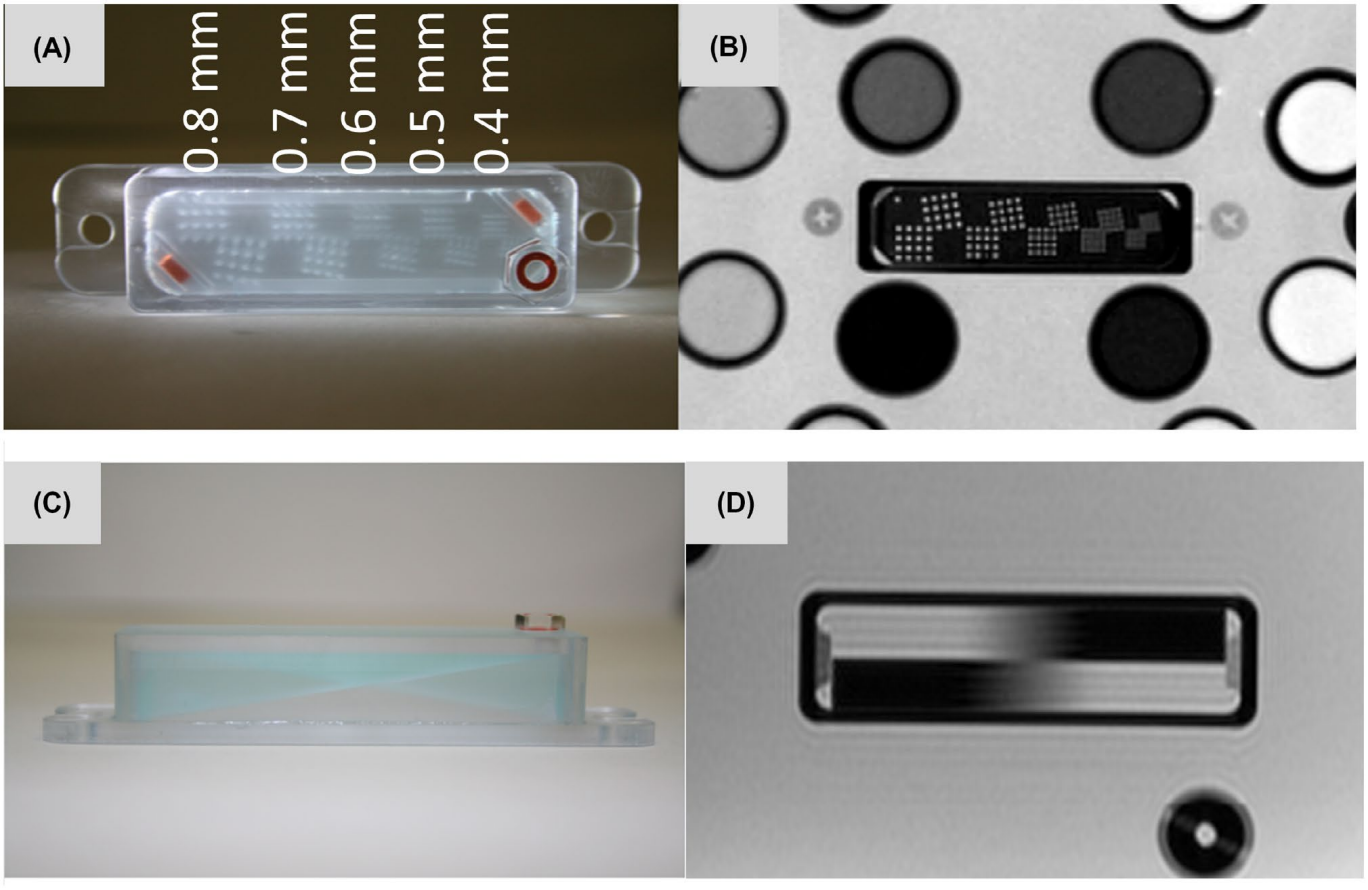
A,B, Photo and MRI of resolution inset. C,D, Photo and MRI of slice profile inset.
